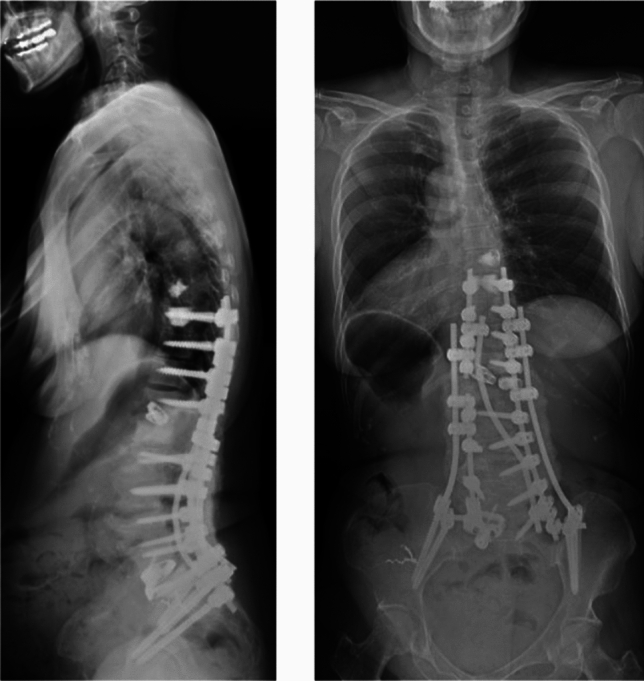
Post-operative radiographs demonstrated improved and acceptable regional and global coronal and sagittal spinal alignment
